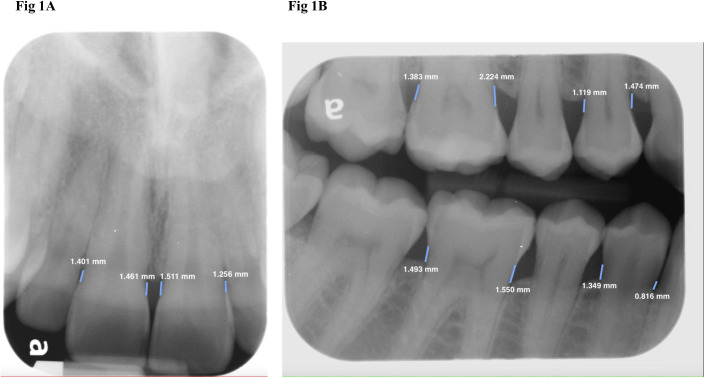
Cementoenamel junction (CEJ) to alveolar crest distance measurements on intraoral radiographs. A. CEJ–alveolar crest distance measurements for maxillary right and left central incisors on periapical radiographs. B. CEJ–alveolar crest distance measurements for maxillary and mandibular first premolars and first molars on bitewing radiographs. The units of measurement are displayed in mm.
